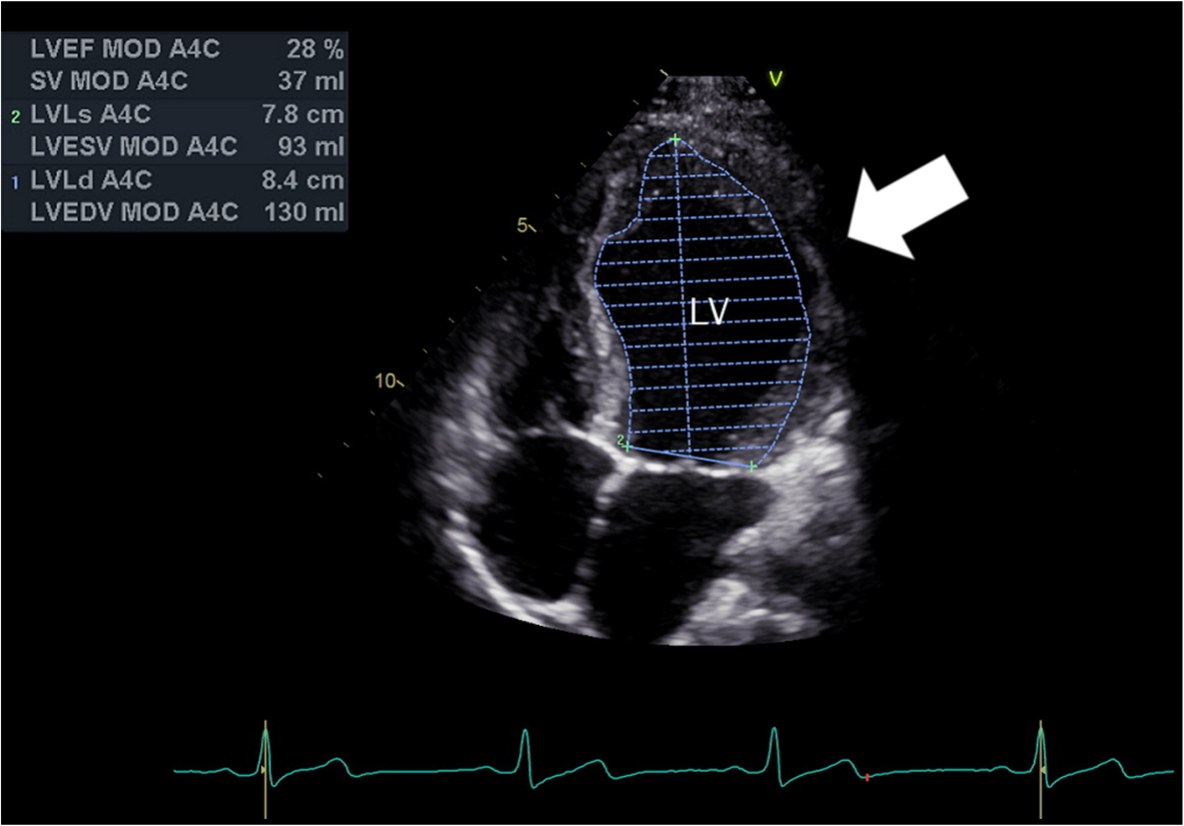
Echocardiogram demonstrating systolic dysfunction with a left ventricular ejection fraction of 28%
